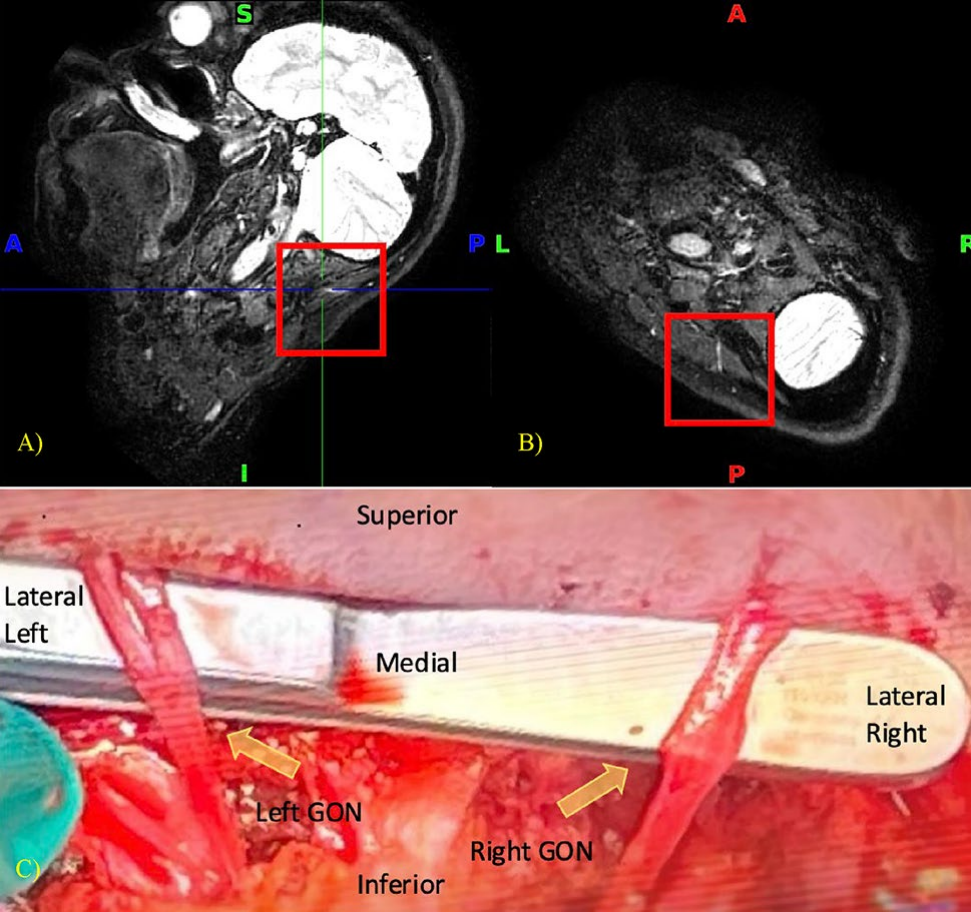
(A) Sagittal and (B) Transverse BrainView MRI showing a split structure suspected to be the GON (red box). (C) Intraoperative image showing the splitting in the left GON (yellow dotted lines) and the absence of splitting in the right GON.
